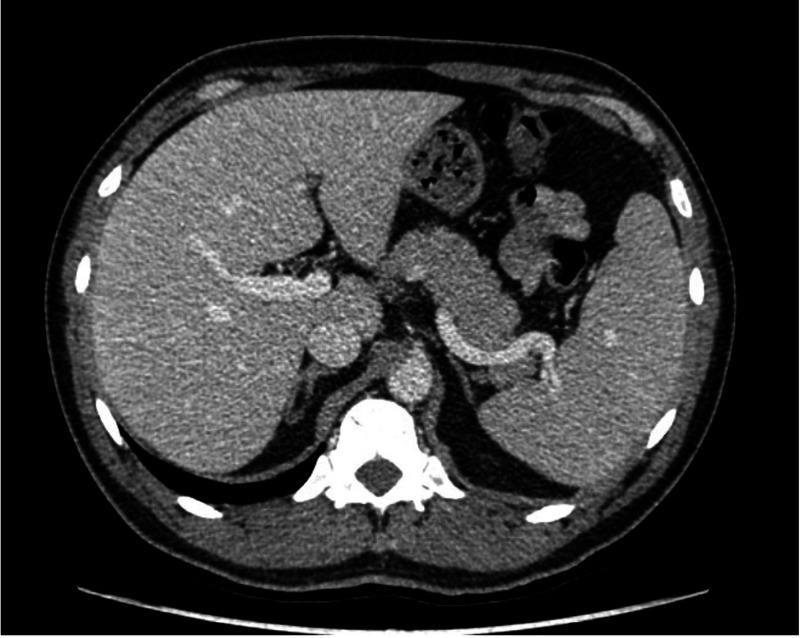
Computed tomography scan showing an enlarged liver and spleen
